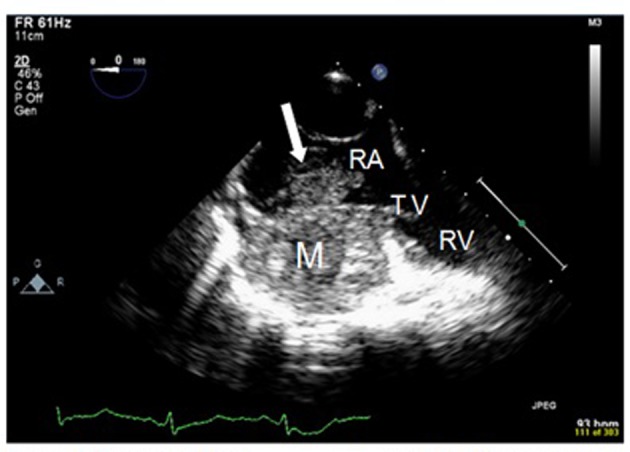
Transesophageal echocardiogram (TOE): transverse view of the right chambers. There is a large firm infiltrating mass (M) in the right atrium (RA), with a propagation (arrow) within the right atrial cavity. RA: right atrium; TV: tricuspide valve; RV: right ventricle.
